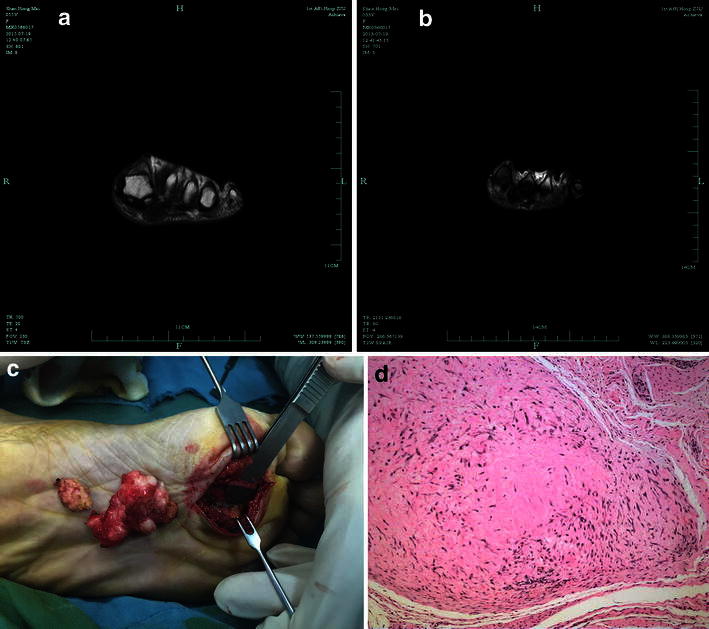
a The tumor was low signal intensity on T1-weighted images, located in the distal planta before the operation. b The tumor was low signal intensity on T2-weighted images before the operation. c The tumor was adhered with the common plantar digital nerve. d For the deep type, the lesion had a lobulated appearance. Every lobulated one was separated with the narrowed and lacunar infarction, around which the remaining tendon sheath structure could be seen. It constituted discrete fibroblast, the confined lacuna vasorum and red collagen fibers. The fibroblast had the shape of short shuttle, the partial cells were rich with bundles of spindle-shaped arrangement (original magnification ×100)
